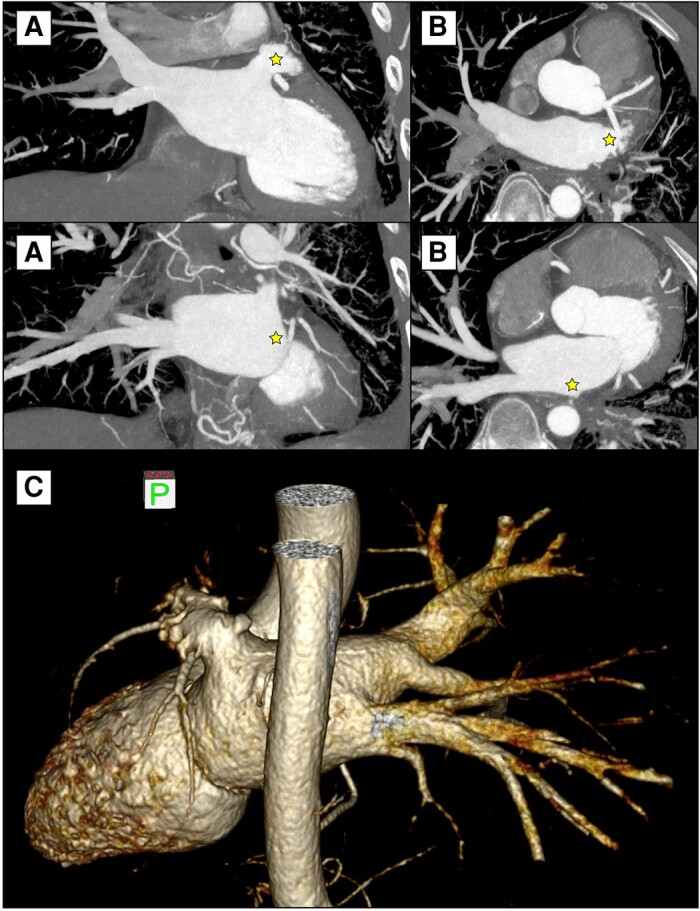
Computed tomography scans of the coronal (*A*) and transverse (*B*) sections showing interruption of the left upper pulmonary vein and absence of the left inferior pulmonary vein (star). (*C*) Posterior view of the 3D reconstruction of the left atrium, left ventricle, and aorta.
